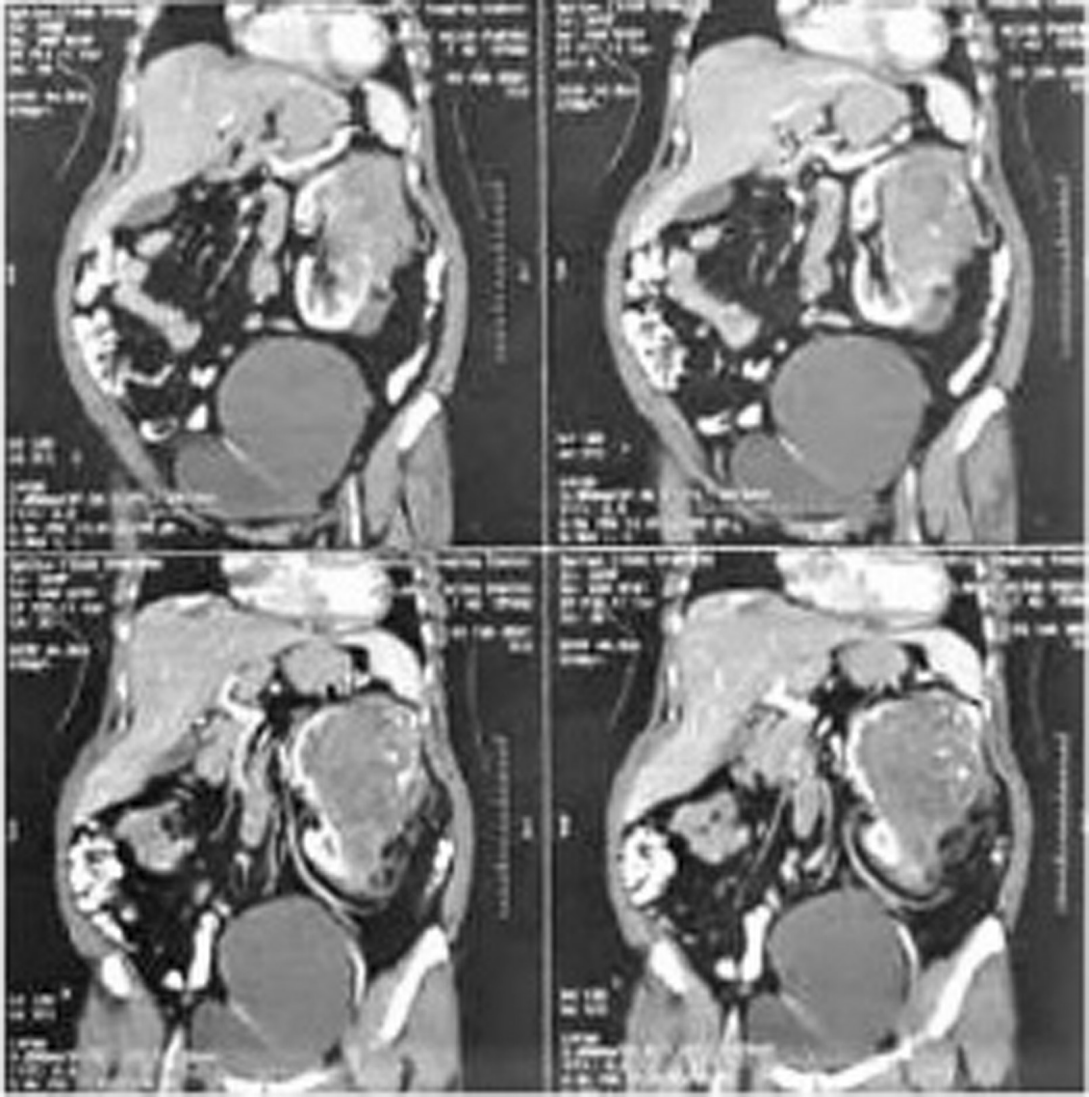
CT Urography showed a large mass originating from the kidney
